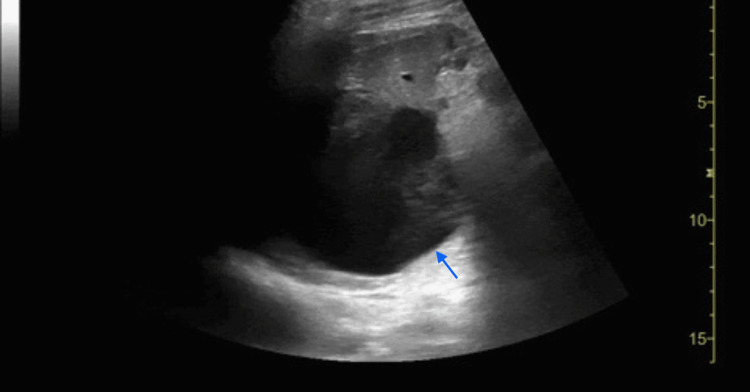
LUQ abdominal ultrasound showing a large cystic structure within the spleen. The cystic structure is identified in the spleen by a blue arrow. LUQ, left upper quadrant
